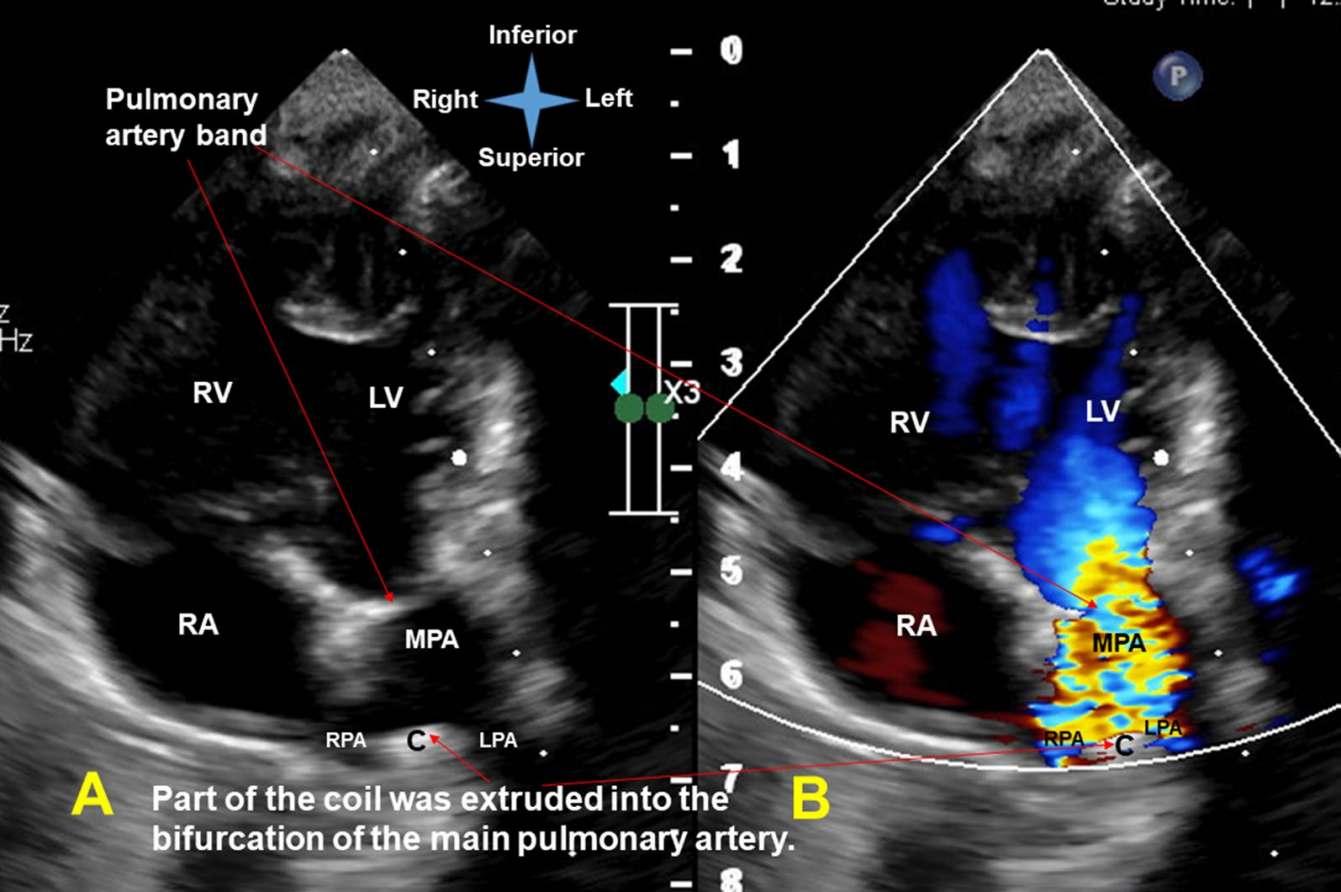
Modified apical five-chamber view (simultaneous 2-dimensional [a] and color flow Doppler [b] recordings) showed no residual ductal shunting, but part of the coil was extruded into the bifurcation of the main pulmonary artery, in addition to the high-velocity mosaic pulmonary flow jet resulting from the previously performed pulmonary artery banding. It also showed the direct collision of the high-velocity pulmonary flow jet with the extruded coil. *C* coil; *LPA* left pulmonary artery; *LV* left ventricle; *MPA* main pulmonary artery; *RA* right atrium; *RPA* right pulmonary artery; *RV* right ventricle
